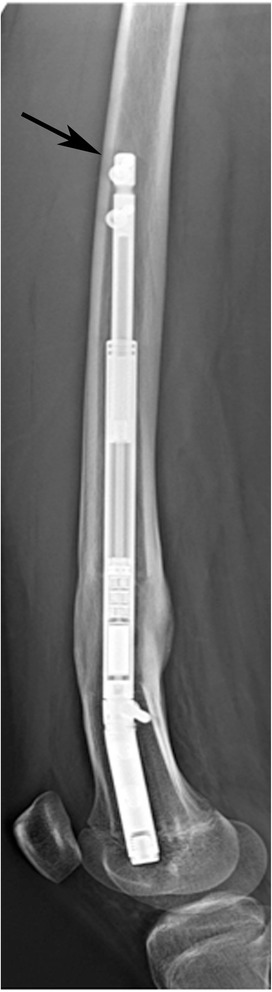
Lateral X-ray showing nail tip impingement on the anterior cortex in the proximal femur
